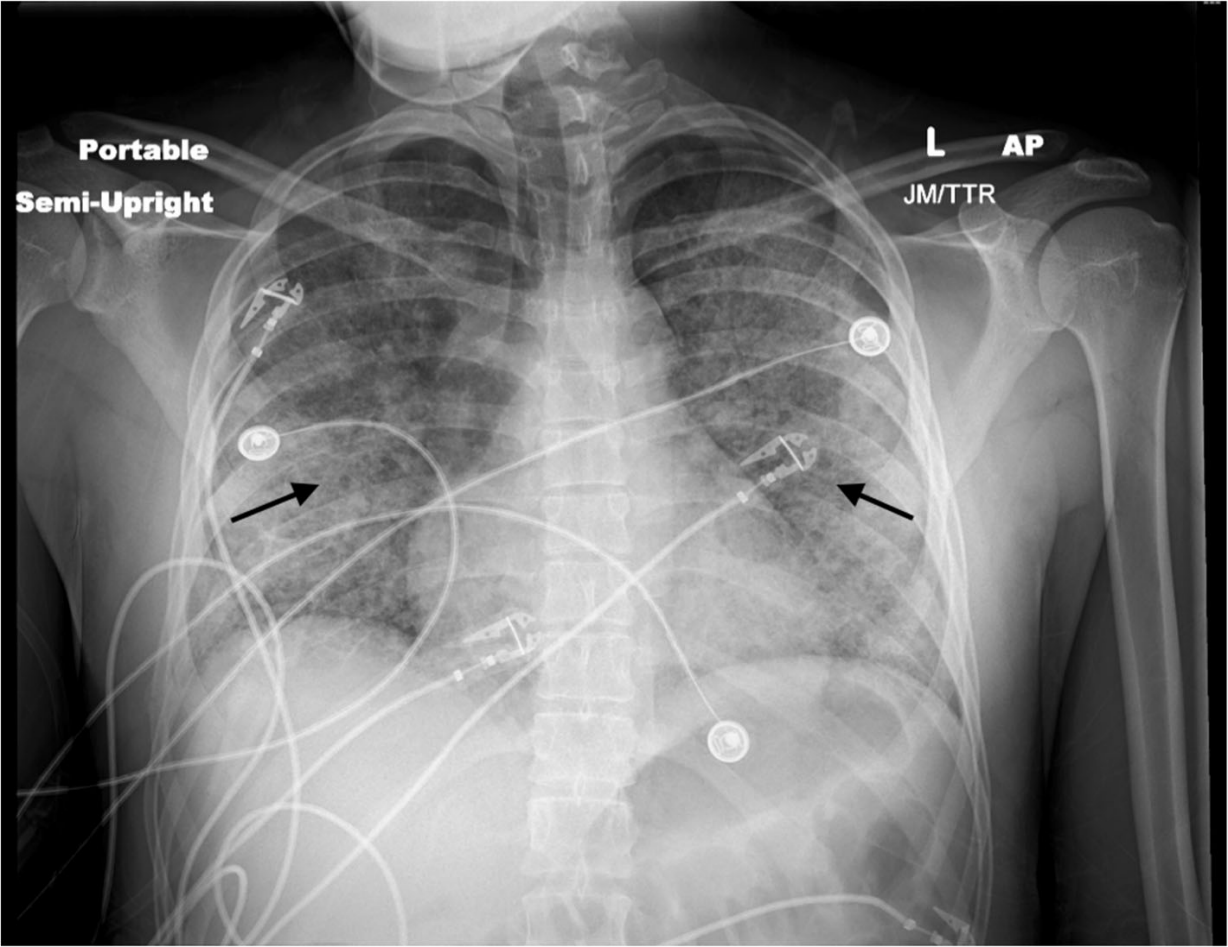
Chest x-ray demonstrating diffuse central predominant interstitial opacities (*black arrows*)
